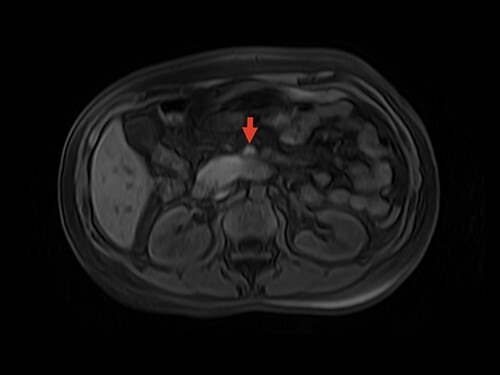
MRI showing superior mesenteric vein thrombus. Arrow: superior mesenteric vein with thrombus inside the vein lumen.
